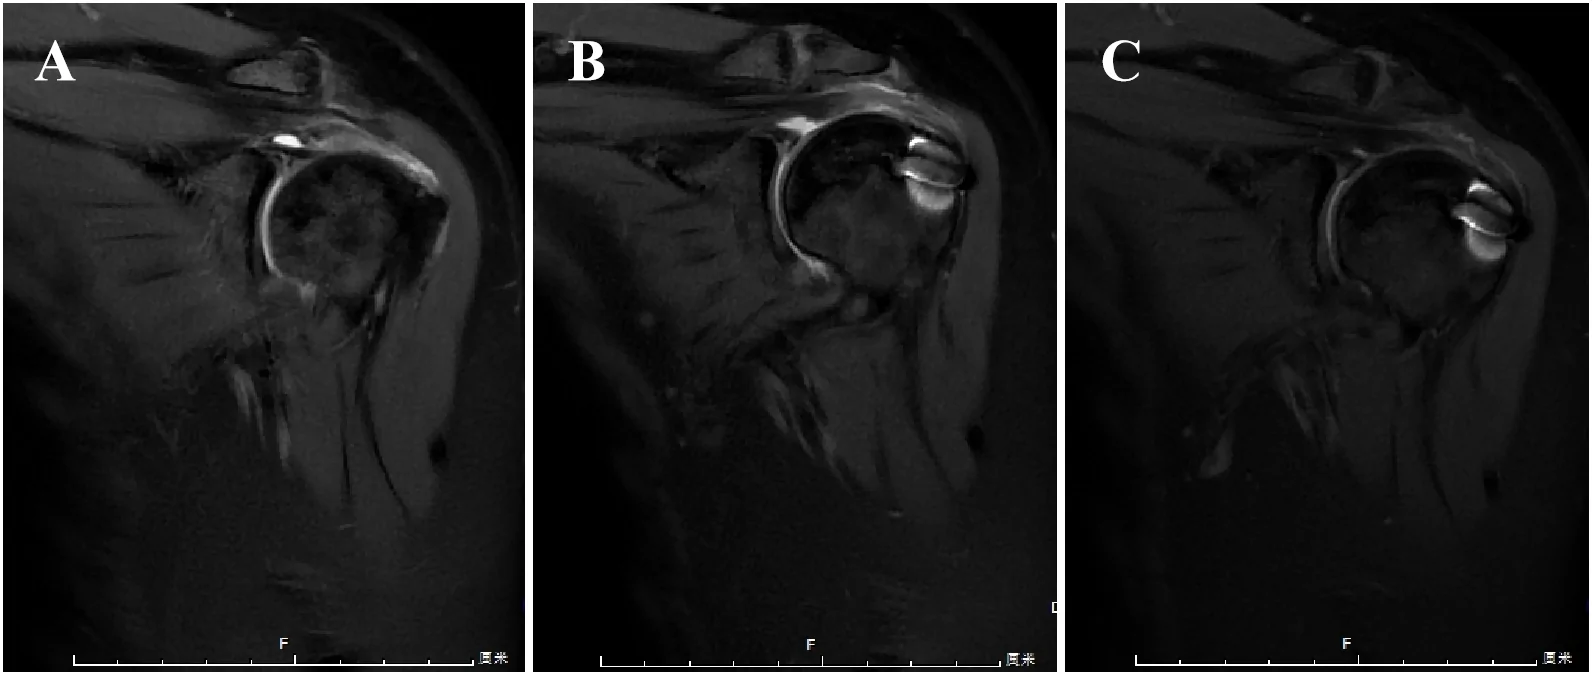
Follow-up assessments of MRI were conducted preoperatively (A), at 6 months (B) and 24 months (C) postoperatively.
